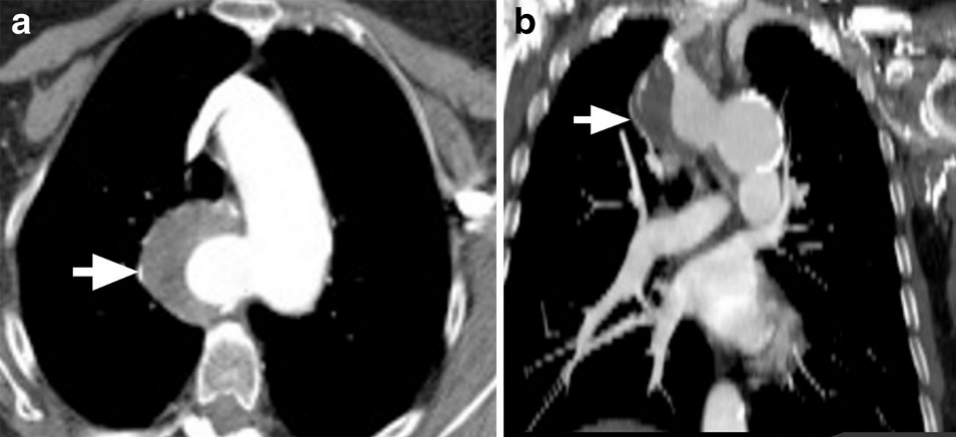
Aneurysm of an aberrant right subclavian artery. a Axial CT scan shows a large aneurysm with partial thrombosis (*arrow*) at the origin of an aberrant right subclavian artery in a patient with a left aortic arch. b Coronal CT reformatted image in the same patient shows the partially thrombosed aneurysm (*arrow*) at the origin of an aberrant right subclavian artery
